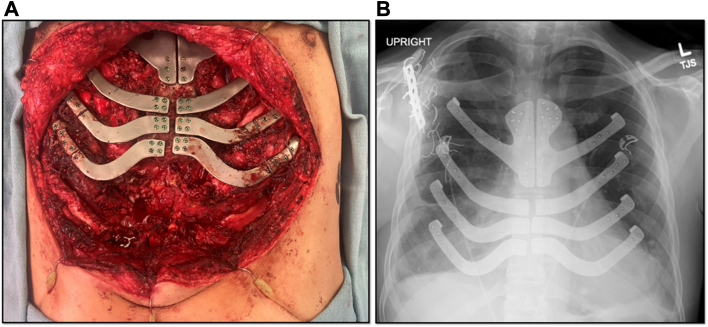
Final sternal plating. (A) Intraoperative view. (B) Chest radiograph on discharge.
